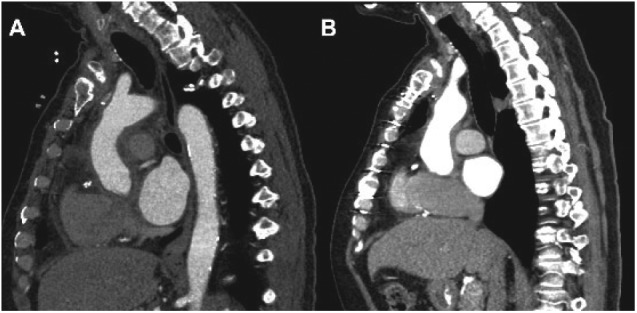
CT angiograms of the chest 1 month (A) and 1 year (B) after intervention.
